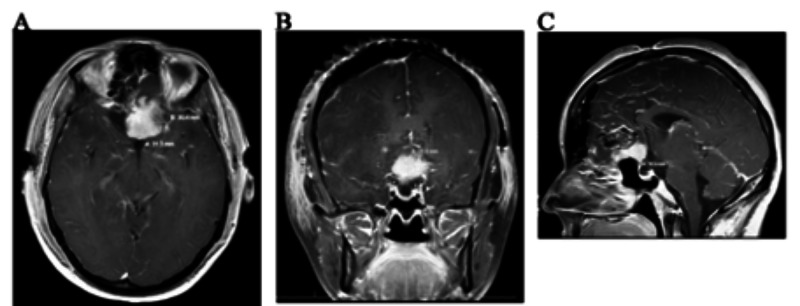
Post-Op CT Post-operative contrast-enhanced T1 axial (A), coronal (B), and sagittal (C) magnetic resonance imaging with residual mass in the anterior cranial fossa superior to the sella turcica.
